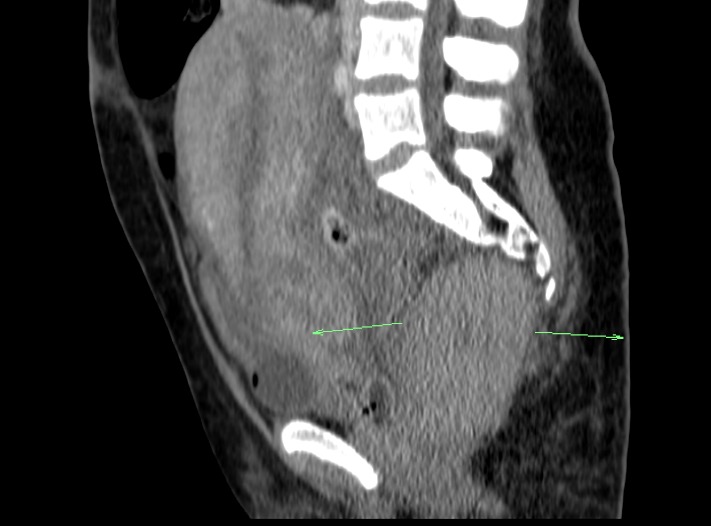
CT image of retro rectal and pelvic retroperitoneal hematoma fused from a perineal laceration associated with childbirth
